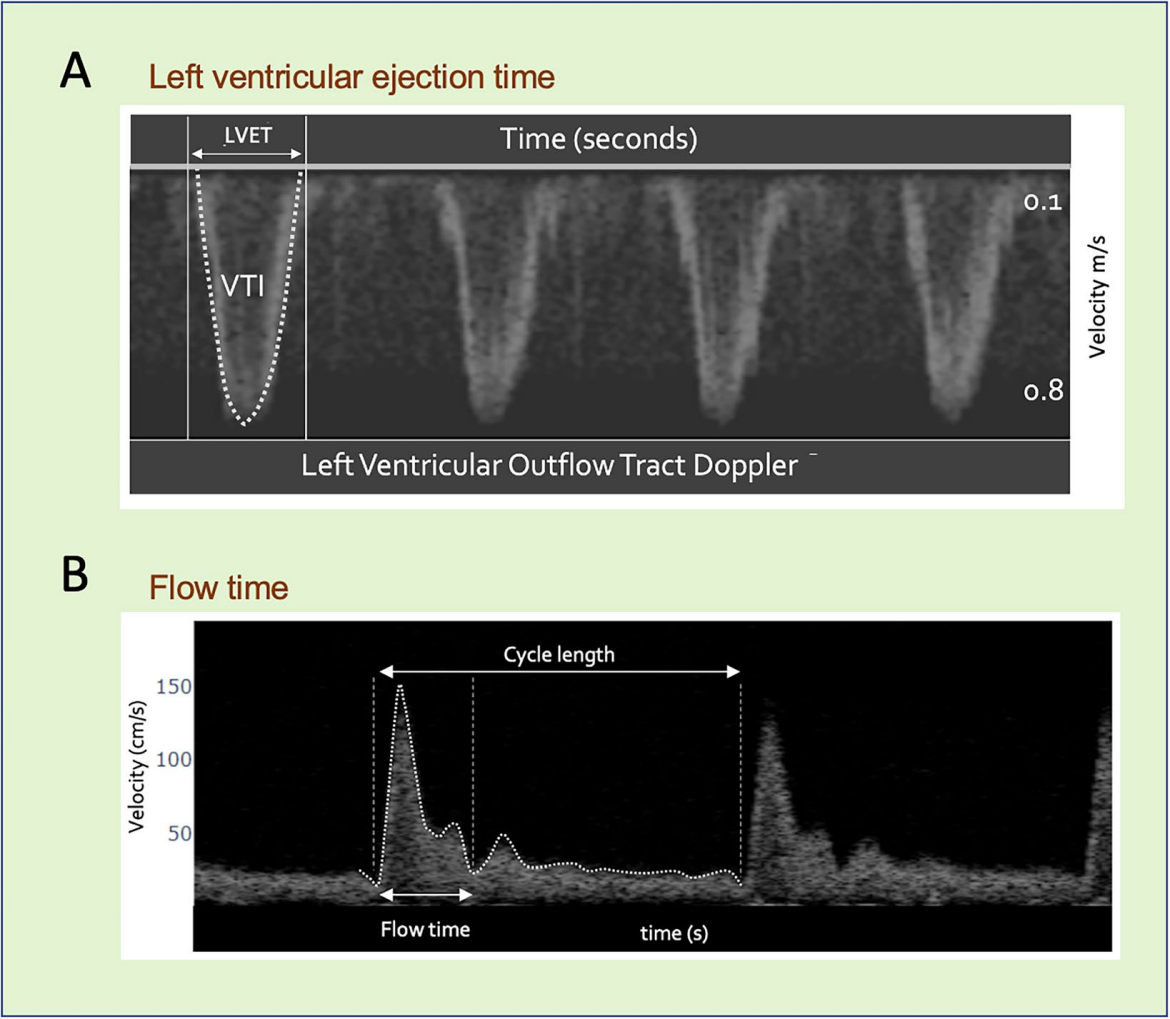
The left ventricular ejection and flow times. A) 4 cardiac cycles obtained via trans-esophageal echocardiography. Velocity increases in the downwards y-axis and x-axis is time. LVET is the duration the aortic valve is open and ejecting blood, the left ventricular ejection time. VTI is velocity time integral. B) Flow time from the common carotid artery. Velocity increases upwards on the y-axis and the x-axis is time
